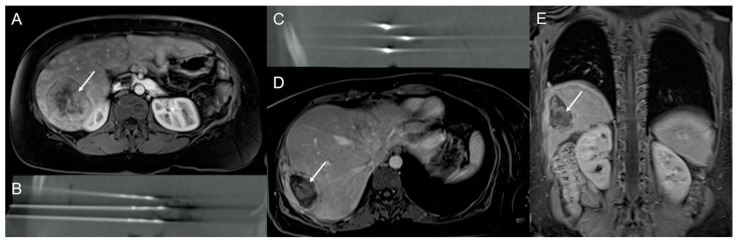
A 39-year-old woman with multiple liver metastases from a grade 2 neuroendocrine tumor that previously underwent systemic treatments. (A) Axial contrast-enhanced T1-weighted MRI image showing a single metastasis of 6.5 cm (arrow) of the right liver lobe, which was not responsive to previous therapies. According to a multidisciplinary board recommendation, tumors were treated by microwave ablation performed with a multiantenna approach (B,C), complicated by postablation bleeding and local infection treated by percutaneous embolization and drainage, respectively. Axial (D) and coronal (E) contrast-enhanced T1-weighted MRI images obtained at the 9-month follow-up showing a shrunken residual necrotic area (arrows).
